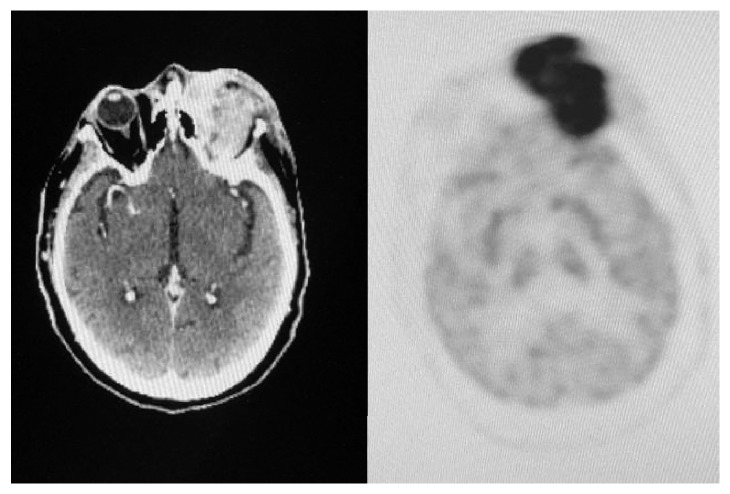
The FDG-PET/CT scan at relapse showed a huge tumor mass with high metabolic rate.
